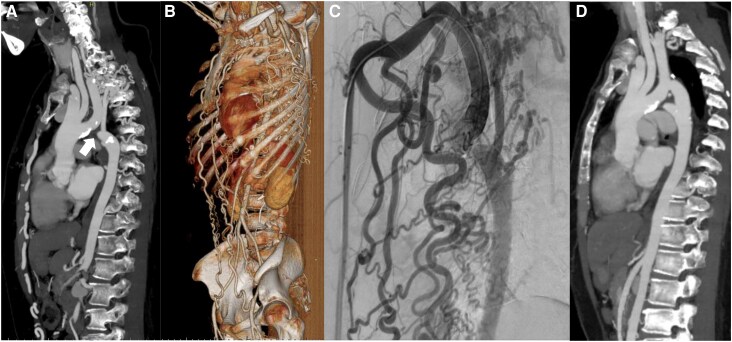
Blood supply to the lower extremities was maintained through collateral channels originating from the bilateral internal thoracic arteries (*Panel B*). Aortography demonstrated opacification of the distal aorta without contrast delay, owing to abundant collateral blood flow from the left subclavian artery (*Panel C*). Transoesophageal echocardiography revealed no patent ductus arteriosus or other congenital anomalies.
